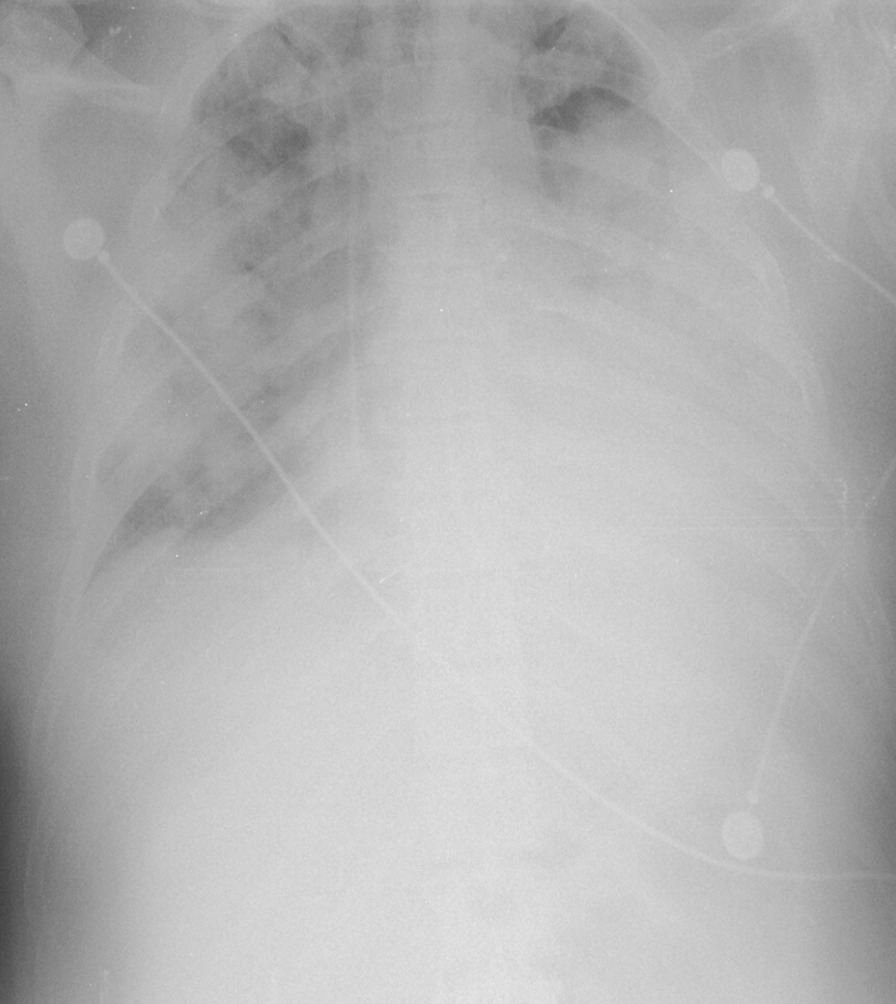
Initial X-Ray in supine body position. The choice of lateral tilt was based on the initial X-Ray that was taken in supine body position. The targeted lateral position strategy was defined by selecting the less aerated lung to be positioned up and the more aerated lung to be positioned down. Please note the left-to-right lung asymmetry present on this initial X-Ray: unequivocally more opacities within the left lung
